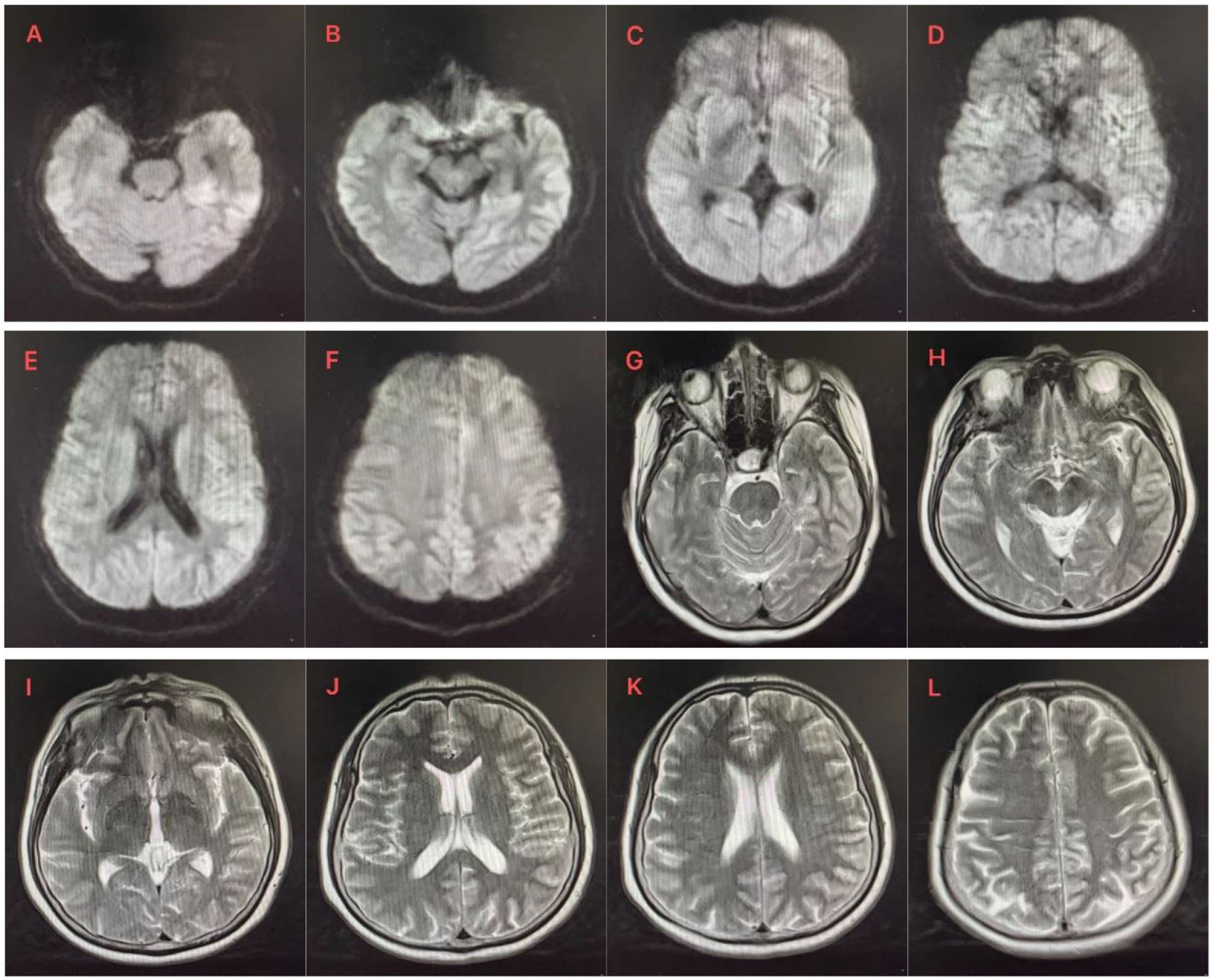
(A–F) DWI showed asymmetric cortical hyperintensity in the bilateral caudate nucleus, bilateral cingulate gyrus and frontoparietal, temporal and occipital gyrus, especially in the left. (G–L) Small patchy long T2 signal shadow was observed next to the posterior horn of the left lateral ventricle. There was no abnormal signal shadow in the remaining bilateral cerebral hemispheres, cerebellum, and brain stem, no abnormal morphological structure was found, the ventricular system was equal and symmetrical, the sulci cistern fissure was not significantly widened, and the midline structure was centered.
